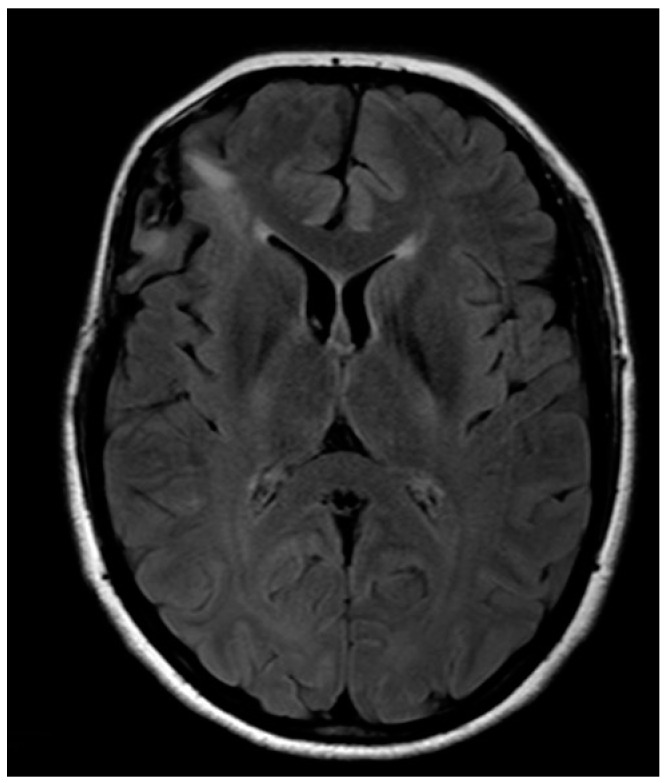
Post-operative anatomical MRI.
